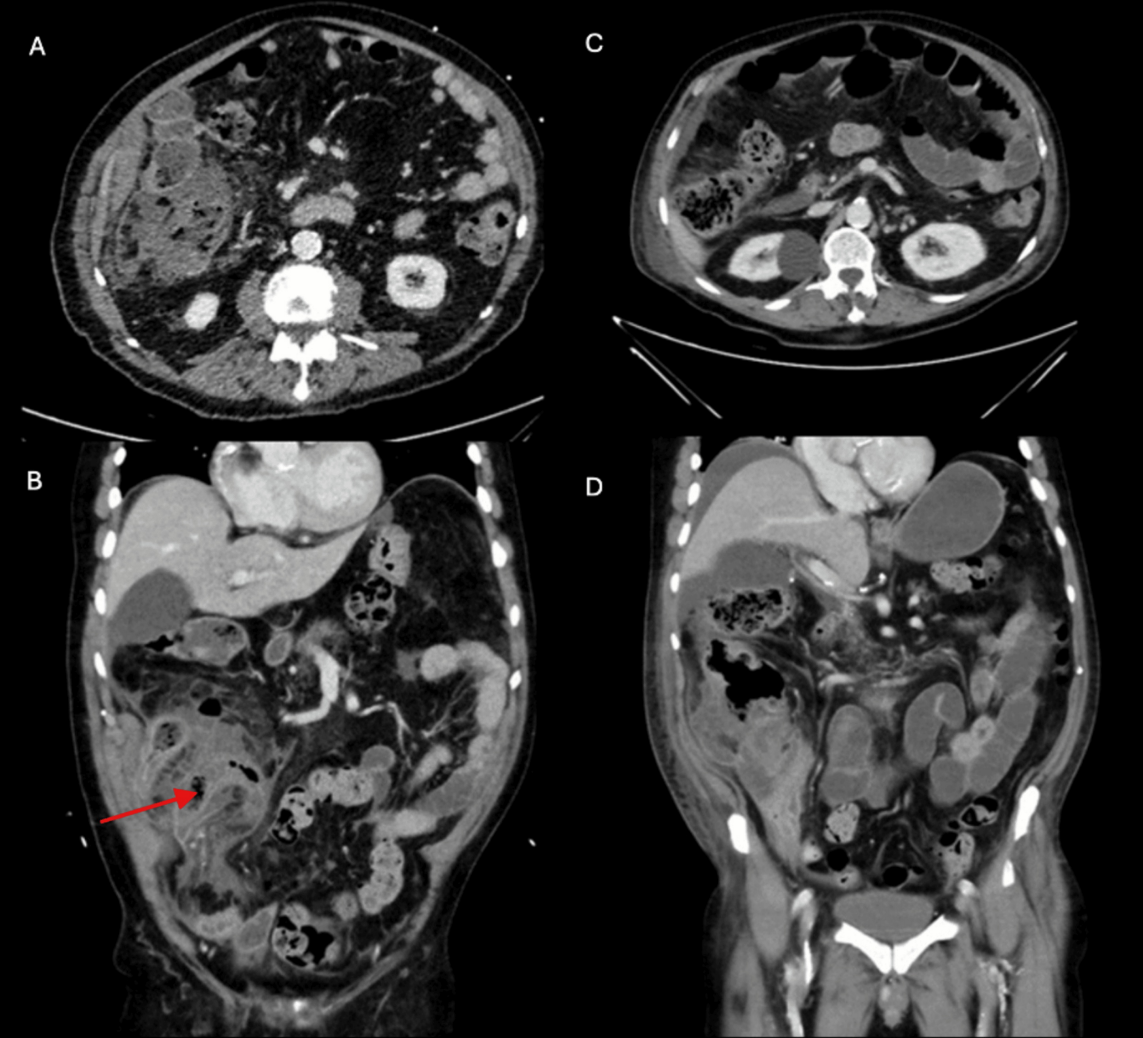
Computed tomography of the abdomen and pelvis with contrast (Case 2). (A) axial section and (B) coronal section on initial presentation, with the red arrow pointing to a markedly thickened appendix measuring up to 25 mm, with extensive inflammatory changes, adjacent fluid densities, and free fluid in the pelvis. (C) Axial section and (D) coronal section of a CTAP performed two days later, demonstrating a collection lateral to the caecum that had perforated, with early signs of small bowel obstruction. There was a suggestion of an underlying caecal malignancy that had perforated. CTAP, computed tomography of the abdomen and pelvis
